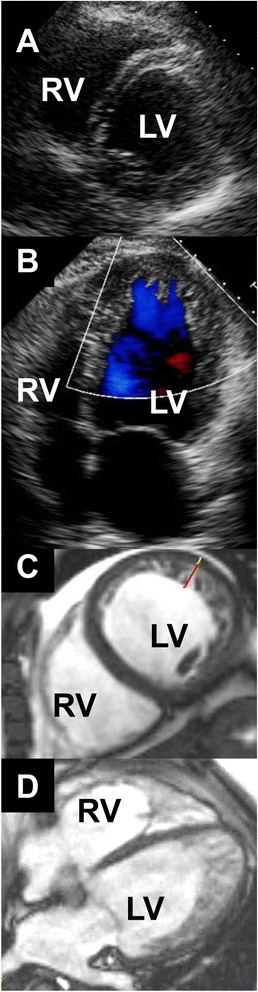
Imaging left ventricular non-compaction in a single patient. A: Short-axis echocardiogram at the level of the chordae tendineae, demonstrating normal-appearing myocardium. B: Apical 4-chamber echocardiogram acquired during early systole, depicting blood flow out of apical sinusoids (blue). C: Short-axis mid-ventricular CMR image, demonstrating abundant trabeculation overlying a very thin compacted myocardial layer in the lateral left ventricular (LV) wall. Red bar: trabecular thickness; white bar: compacted wall thickness. D: 4-chamber CMR image depicting LVNC extending from the apical septum clockwise to the lateral LV wall. RV: right ventricle.
